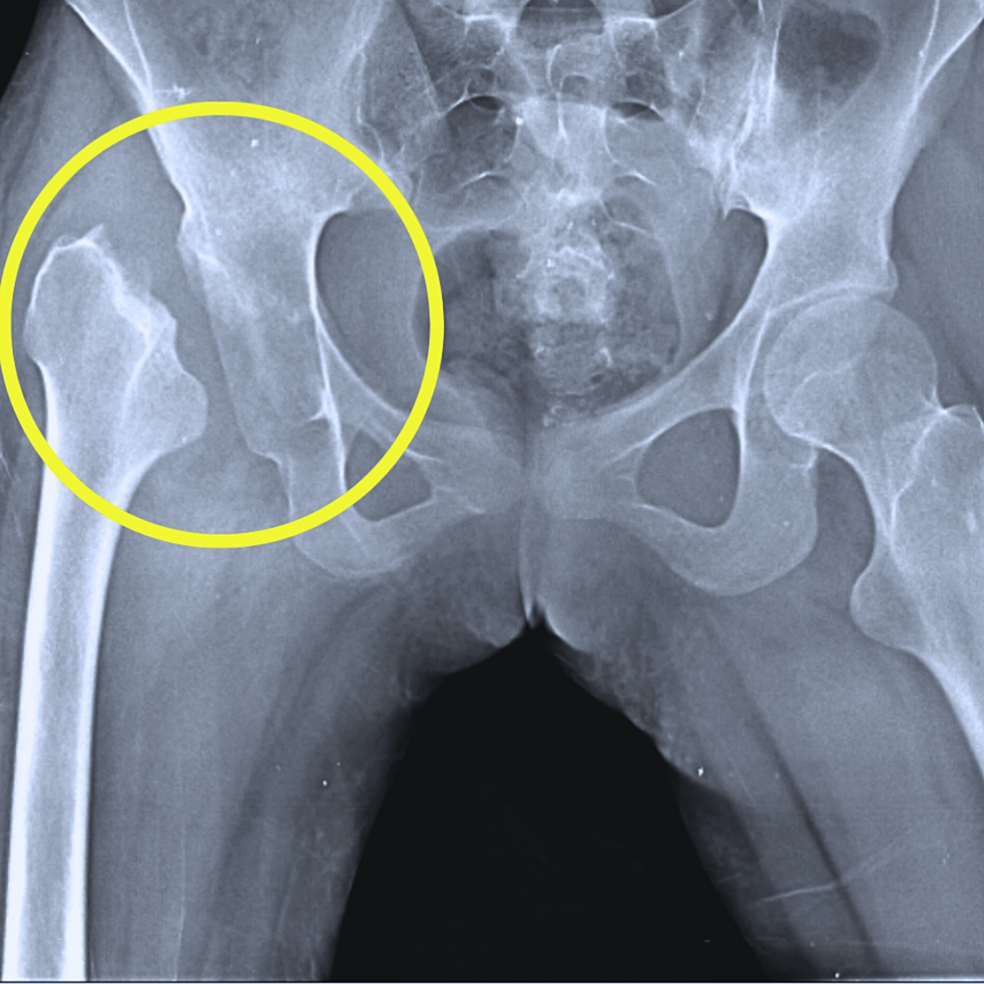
Anteroposterior view of radiograph shows the girdlestone arthroplasty The yellow circle shows the girdlestone arthroplasty.
